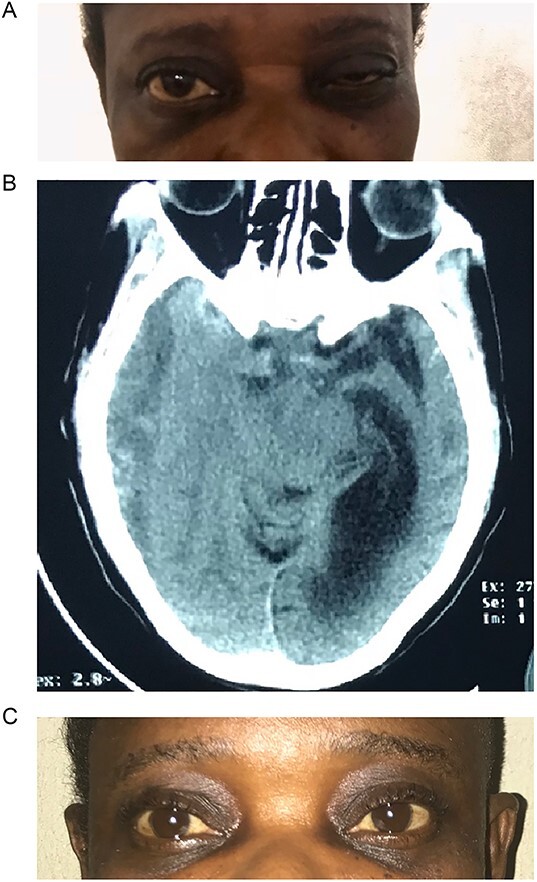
(A) Pre-op with right ptosis/ophthalmoplegia. (B) Pre-op axial CT showing right CSDH. (C) Post op with resolution of ptosis.
